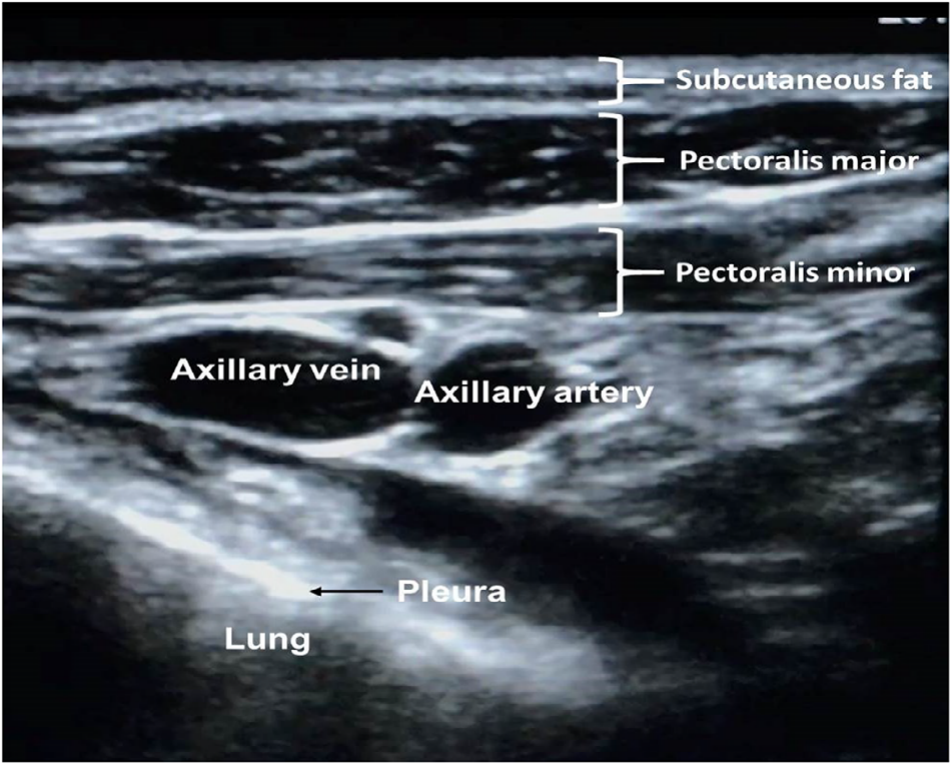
Ultrasound‐guided axillary vein anatomy. Ultrasound images are obtained by placing a vascular probe below and perpendicular to the clavicle. [Colour figure can be viewed at wileyonlinelibrary.com]
